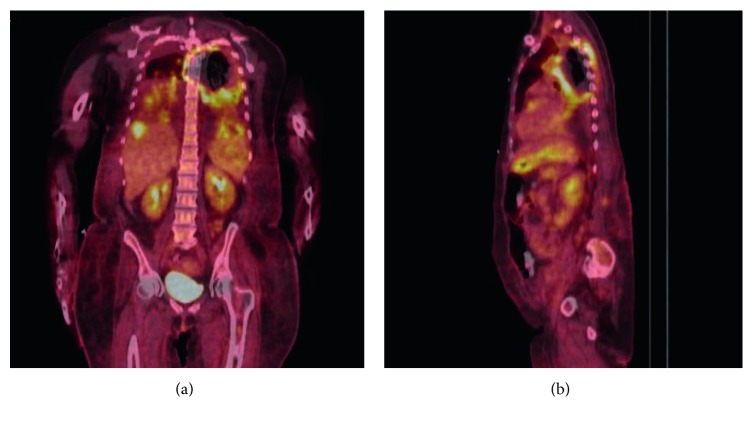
PET/CT images obtained in evaluation of aortic filling defect (coronal image (a); sagittal image (b)), showing hypometabolic activity within the spinal cord from T3 through T6 and a large (6.3 × 6.4 × 5.3 cm), ring-like area of FDG avidity with central, decreased avidity consistent with an abscess and central infarct of the left upper lobe, posterior chest wall, and adjacent thoracic spine vertebral bodies.
